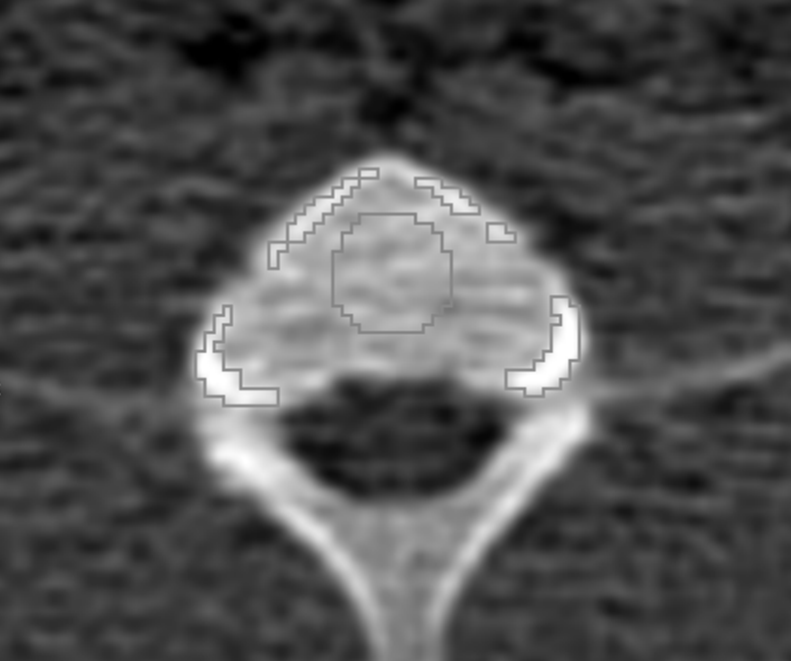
Computer-assisted delineation of the cortical (white) and trabecular (black) bone tissues in the vertebrae. Isocontour ROIs were generated by thresholding on CT images. In this example, the cortical and trabecular ROIs have average densities of 391 and 255 HU, respectively.
